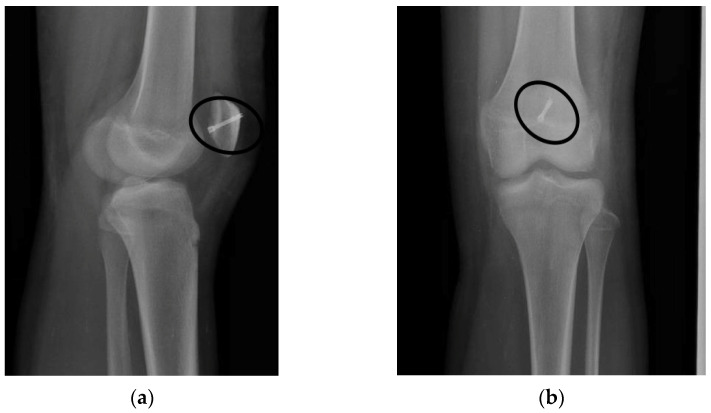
Follow-up X-ray radiographs of the patient in two planes, (a) L view and (b) AP view, of the injured knee. The medical images show that the fracture is healing and put in evidence a good tolerance of the screw by the surrounding tissues.
